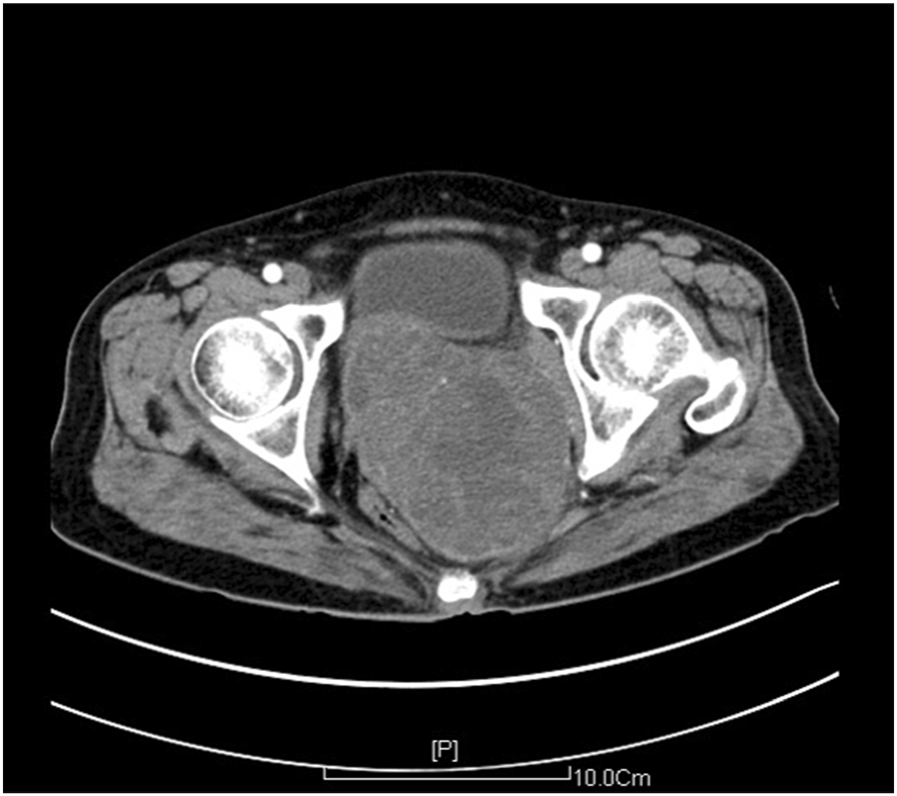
Computed tomography. A mass sized approximately 11.2×9.0cm was detected by computed tomography in the posterior vaginal wall.
